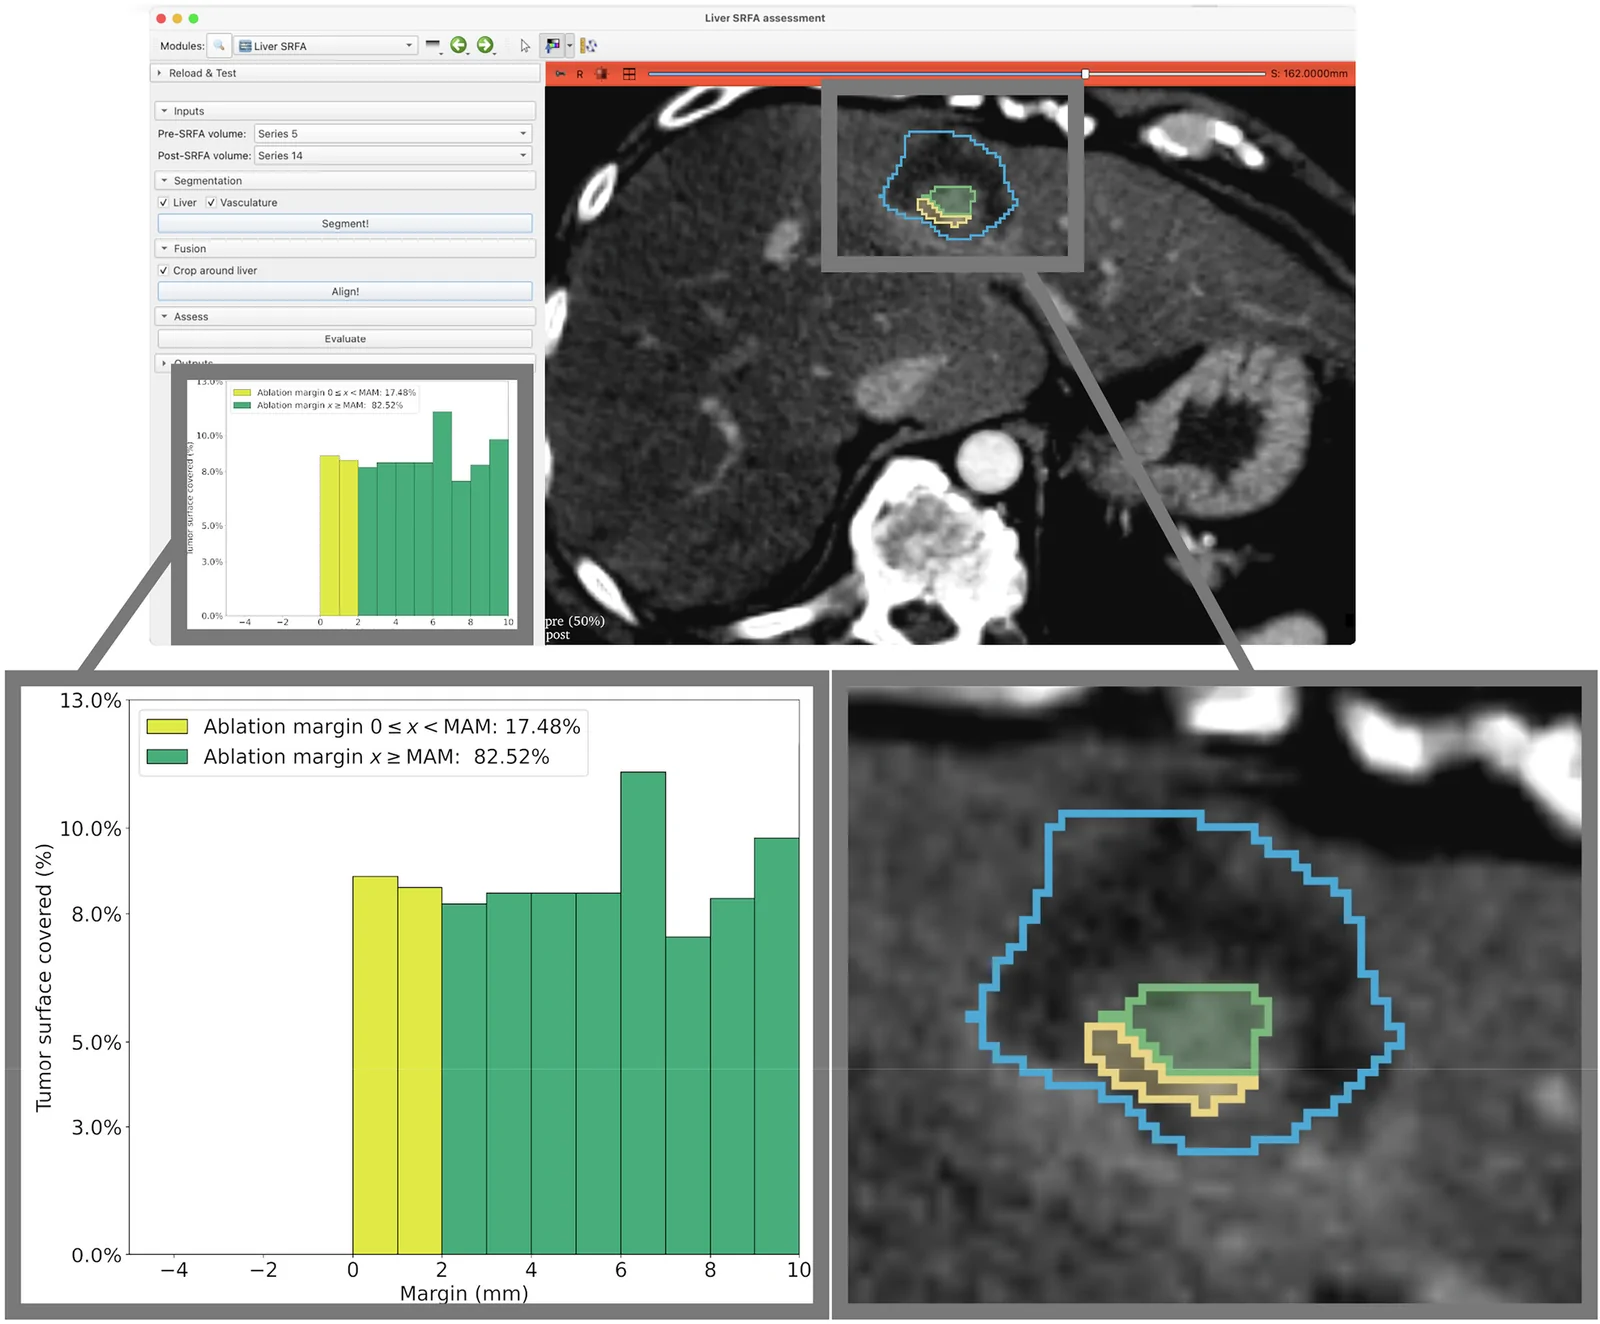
A screenshot of the proposed 3D Slicer extension. Here, we used the blending option to visualize the intraprocedural computed tomography (CT) scans after registration, both with 50% opacity. The outline of the ablation zone is colored in blue. We used yellow and green to mark areas of the tumor with a margin between 0 and 2 mm, and ≥ 2 mm, respectively [see reference [11]]
